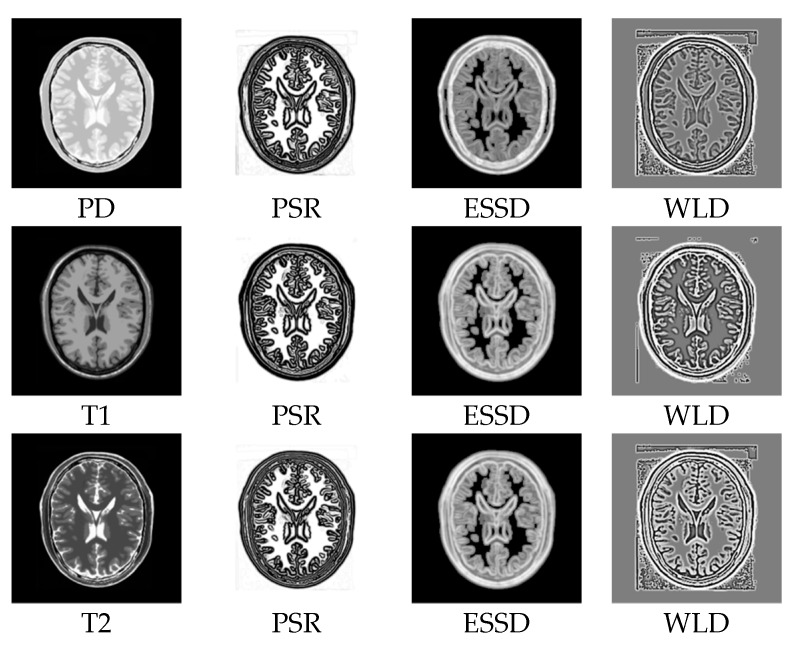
Structural representation results of different MR images. The first column corresponds to the PD-weighted, T1-weighed, and T2-weighed MR images, respectively. The second to fourth columns correspond to the representation results of MR images for PSR, ESSD, and WLD, respectively.
